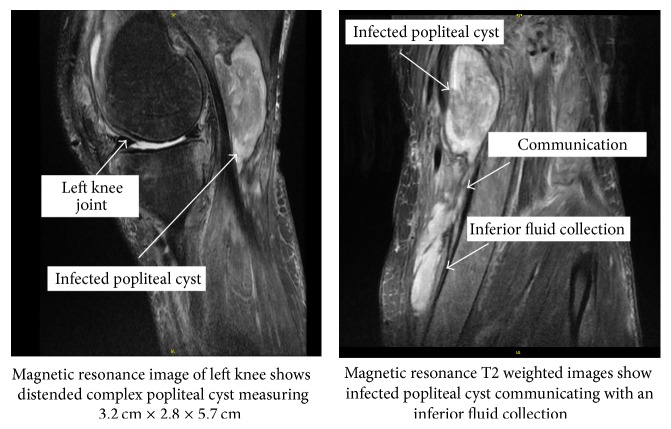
MRI images of left knee and infected fluid collections.
